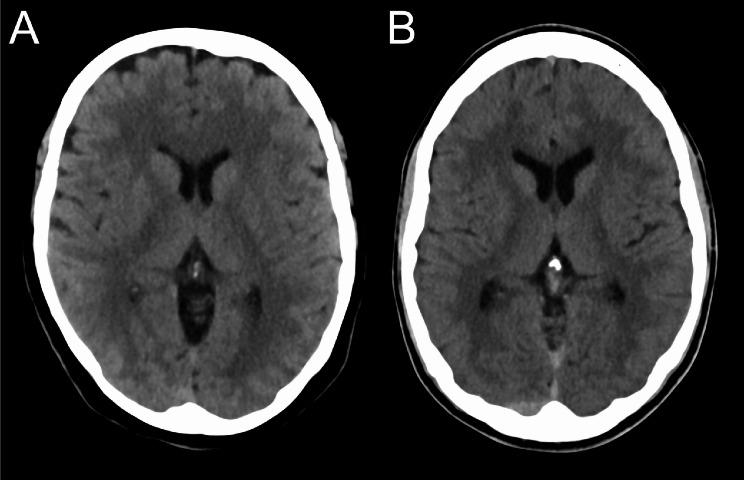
VMI 65 keV in VA50 and VB10 for axial CT images. A: VMI 65 keV in software VA50; B: VMI 65 keV in software VB10. The VMI 65 keV is currently the VMI used in clinical routine and was therefore selected for the qualitative analysis. In this VMI 65 keV, a closer look also reveals that in the VA50A the gray matter directly below the calvarium appears denser than cortical gray matter remote from the calvarium, for example, the cortical gray matter of the insula. Such a difference is subjectively hardly recognizable with the VB10.VMI: virtual monoenergetic image; keV: kilo electronvolt
